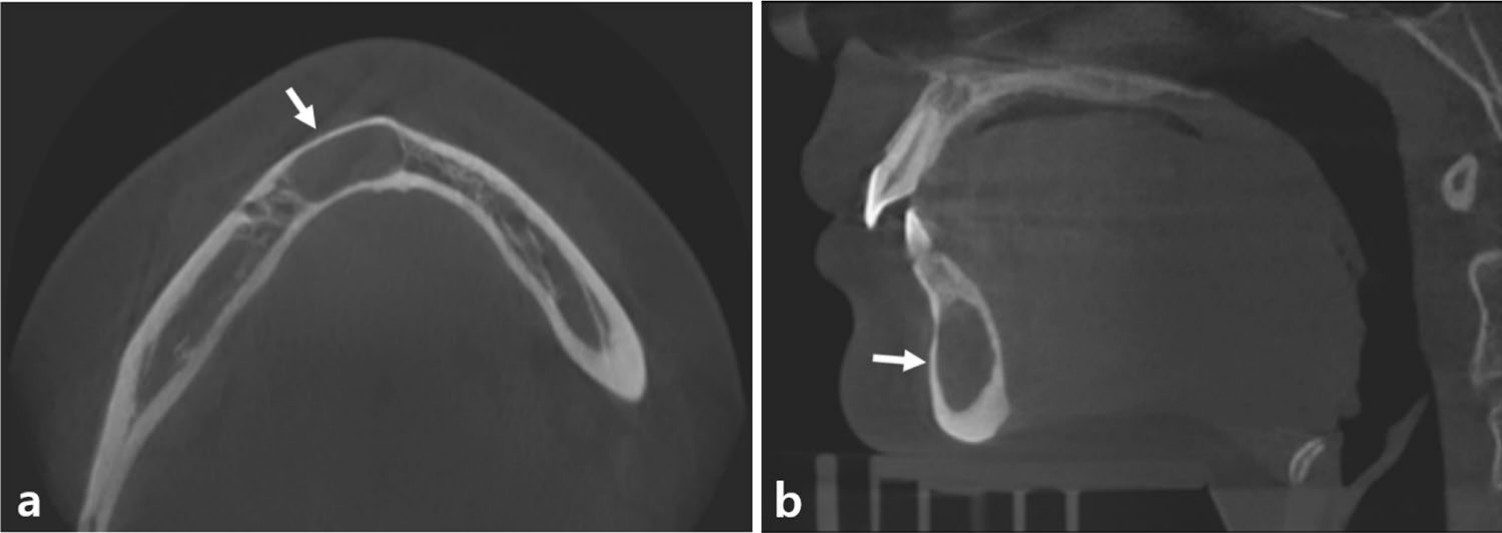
Cone-beam computed tomographic images. Axial (a) and sagittal (b) images show simple bone cyst in the anterior mandible (white arrow). The cavity is entirely filled with the fluid without aeration, and thinning of the buccolingual cortex is observed.
